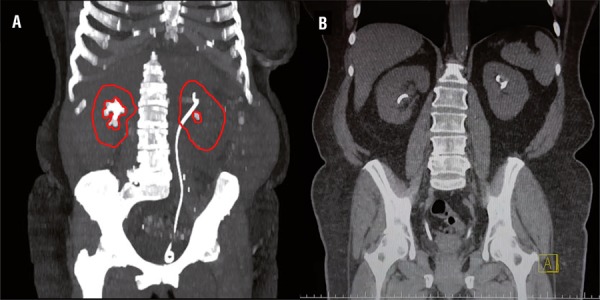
A) preoperative CT scan showing an incomplete staghorn stone in the right kidney of low density and a 10mm calyceal left kidney stone; B) postoperative CT scan showing the stone free status and bilateral ureteral stents.
